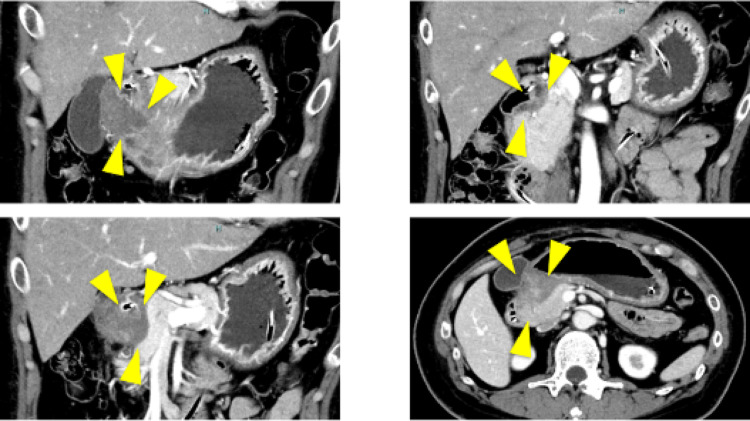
Contrast-enhanced computed tomography demonstrates circumferential thickening around the pylorus and contact with the pancreatic head
